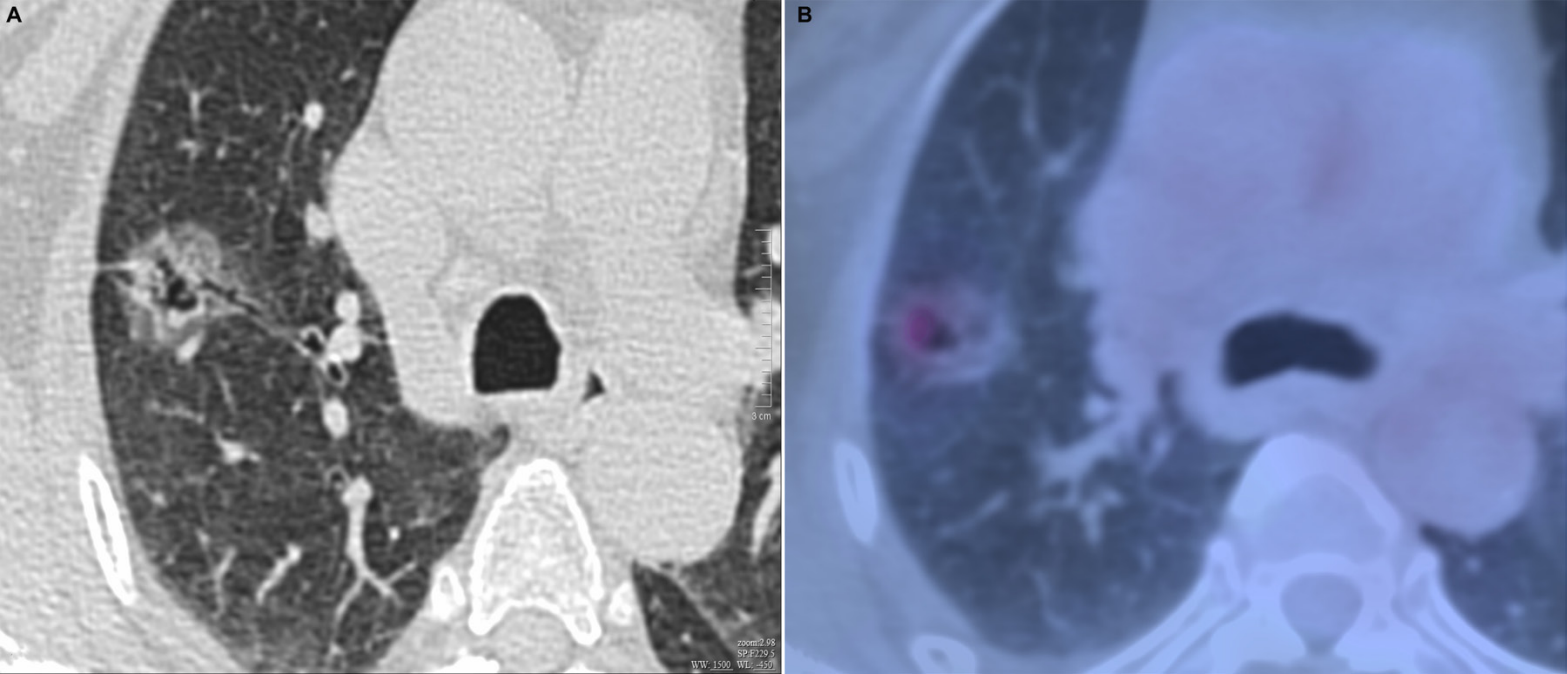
Invasive adenocarcinoma in 58-year-old woman (A) transverse lung-window HRCT scan demonstrates a round, lobulated, well-defined subpleural mixed GGN with natural bronchus sign, cystic appearance, and pleural indentation in the apical segment of right upper lobe. (B) is caudal to (A): PET/CT fusion image with perfusion mode shows a 22.5-mm oval mixed GGN with 0.68 of CTR and 3.32 of SUV_max_.
